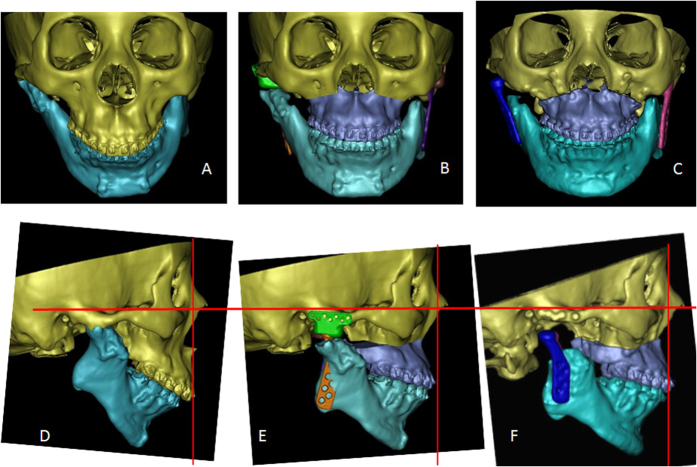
Pre-operative and post-operative CT images of Patient 4. (A) Pre-operative frontal view of 3D CT; (B) Pre-operative design of frontal view of 3D CT; (C) Post-operative frontal view of 3D CT; (D) Pre-operative profile view of 3D CT; (E) Pre-operative design of profile view of 3D CT; (F) Post-operative profile view of 3D CT.
